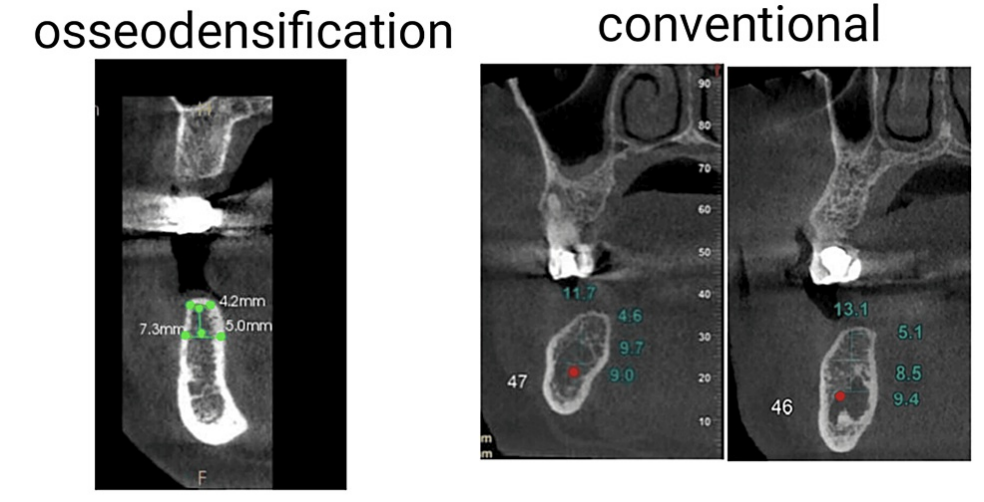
Preoperative cone-beam computed tomography showing narrow alveolar ridge.
